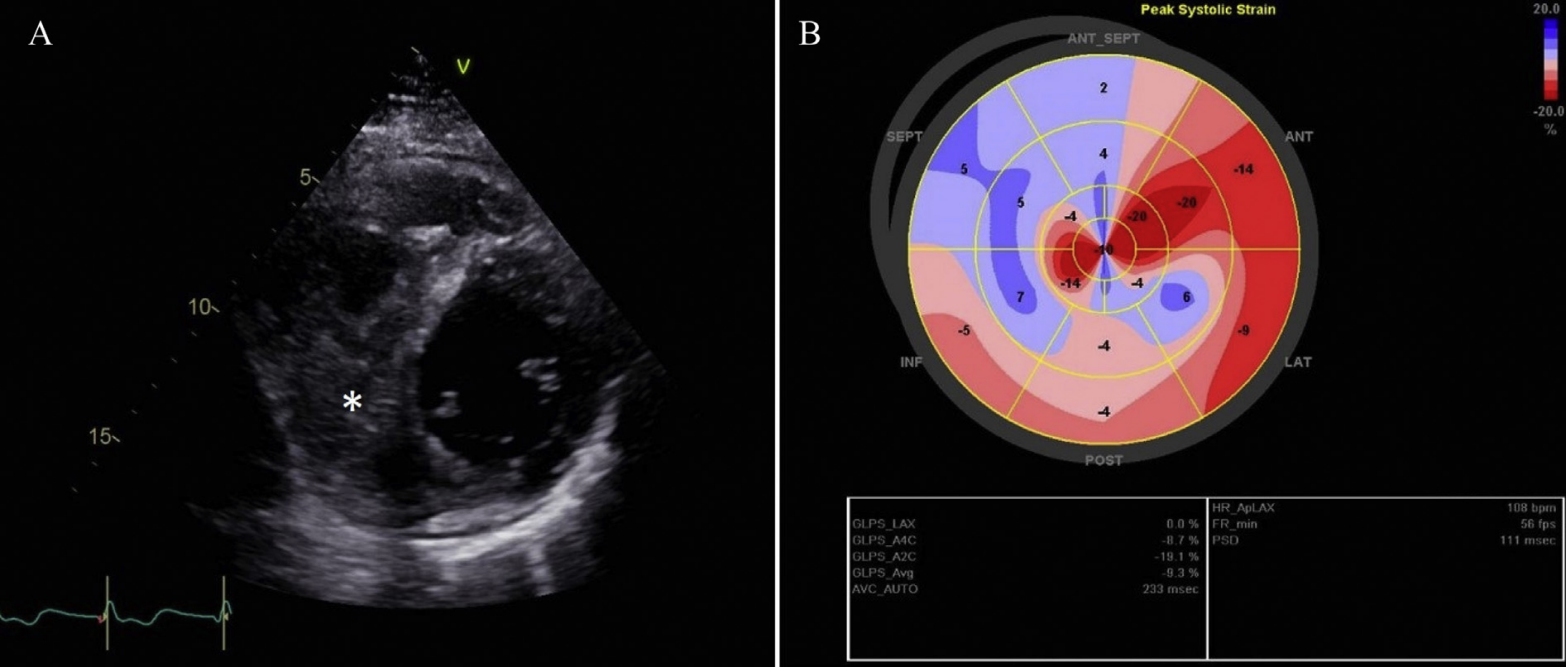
Transthoracic echocardiogram was initially performed due to suspected myocarditis. It revealed a large mass with hypoechogenic areas and a mildly to moderately reduced LVEF (40%) due to hypo- and dyskinesia in the affected areas. (A) The parasternal short-axis view at the papillary muscle level showing the mass in the inferoseptal wall and a minimal pericardial effusion. (B) The bull's-eye plot from the two-dimensional speckle-tracking echocardiography analysis visualizing the regional wall motion abnormalities and a reduced global longitudinal strain of –9%.
